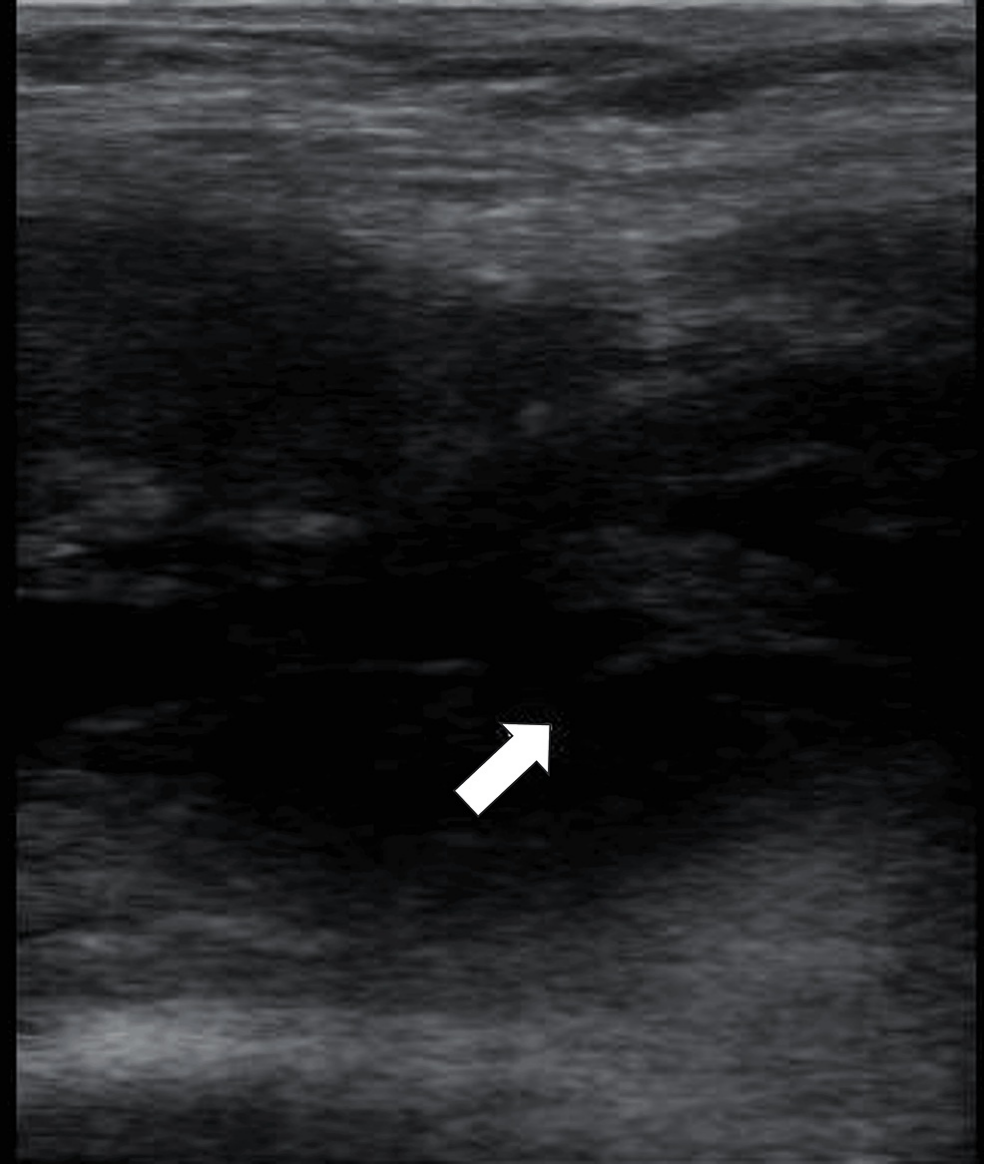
Ultrasound image of a suprapatellar hematoma, showing coagulation debris (arrow)
